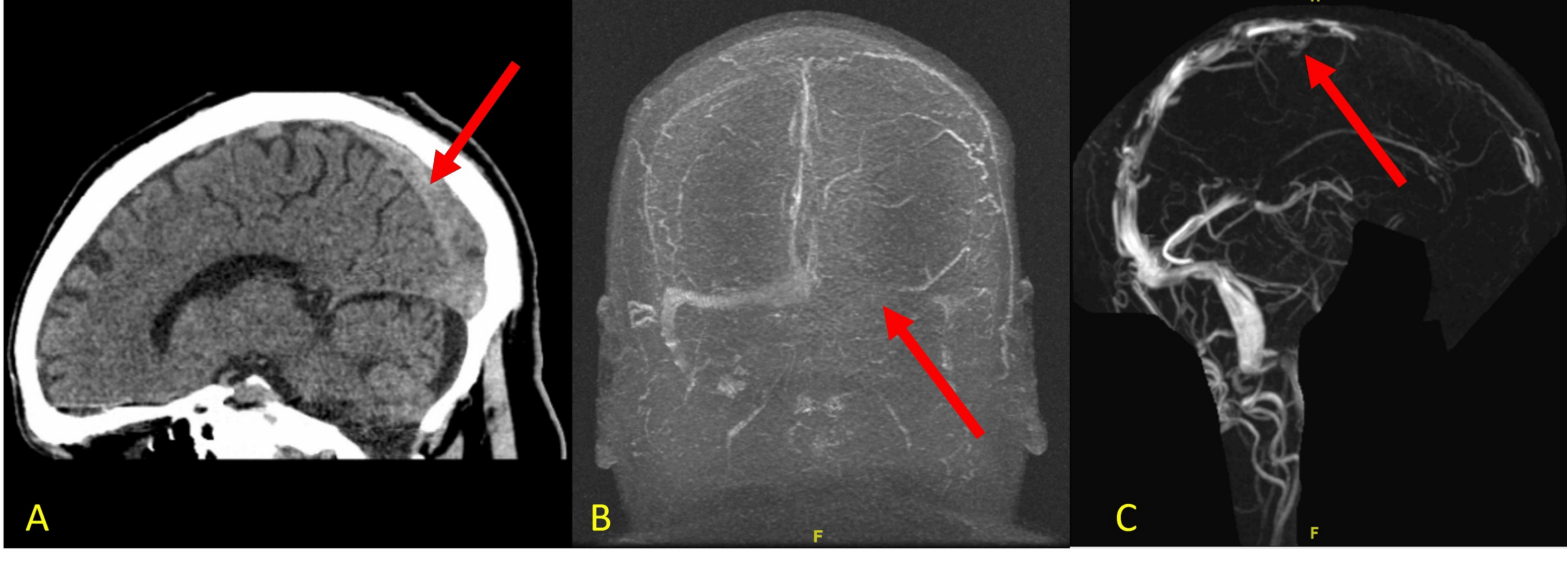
Imaging CT: computed tomography; MR: magnetic resonance (A) CT head showing decreased density within the superior sagittal. (B and C) MR venogram showing dural venous thrombosis in the superior sagittal sinus, extending to the left transverse, sigmoid sinuses, and left vein of Trolard
